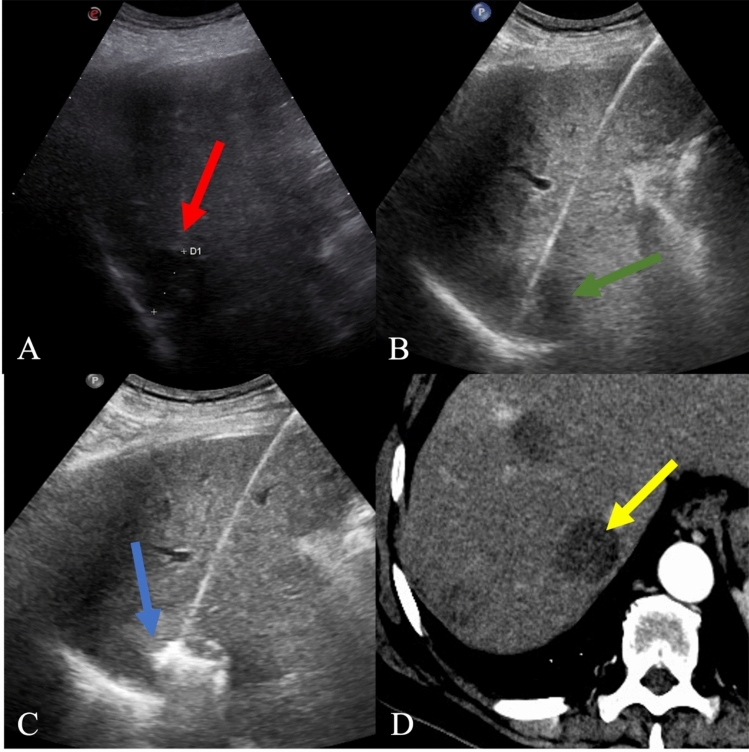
A Hypoechoic HCC (red arrow) of 27 mm in segment VIII, evaluated for percutaneous, US-guided ablation; B Transhepatic microwave antenna (green arrow) with the tip inside the nodule; C Hyperechoic transformation of the nodule (blue arrow) after 8 min of 100W ablation, due to gas bubbles; D 6 months post-operative contrast-enhanced CT scan, demonstrating ablation radicality around necrotic volume (yellow arrow)
